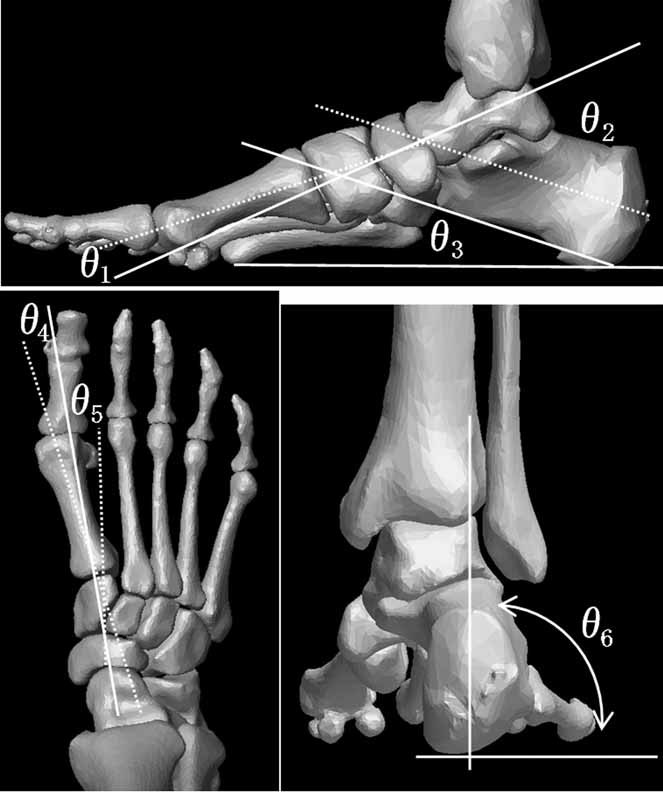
Radiographic views and associated joint angles. Top: Lateral view of the foot showing Talo-1st MetaTarsal (L-T1MT) angle, *θ*_1_; TaloCalcaneal (L-TC) angle, *θ*_2_; and Calcaneal Pitch (L-CP) angle, *θ*_3_. Bottom left: 70° raised AP view of the midfoot showing Talo-1st MetaTarsal (AP-T1MT), *θ*_4_ and TaloNavicular angle (AP-TN), *θ*_5_. Bottom right: posterior view of the calcaneus showing hindfoot varus/valgus measured from the lateral aspect, *θ*_6_.
